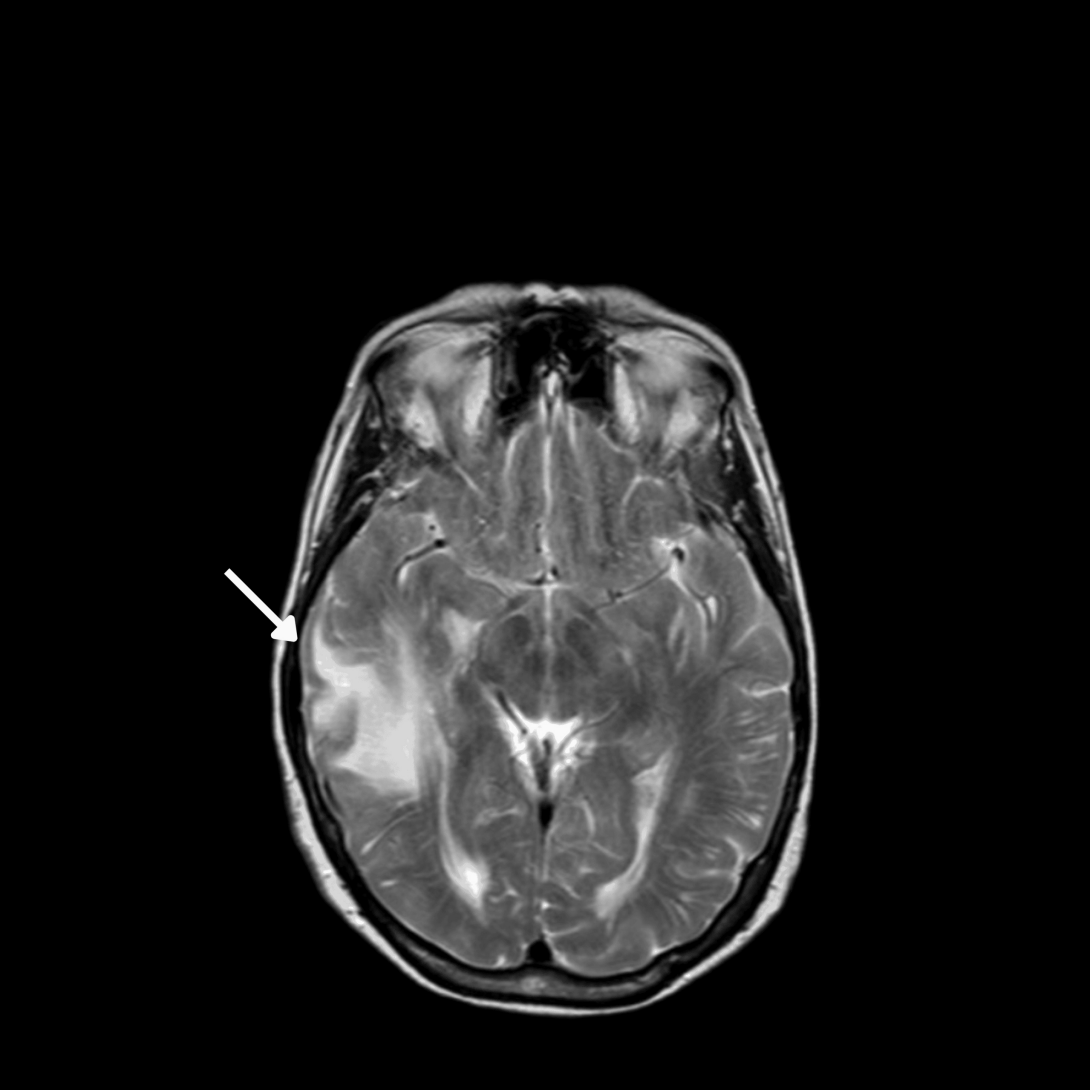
MRI performed in September 2013 showed increased T2 signal (arrow) in the right temporal lobe. MRI, magnetic resonance imaging
